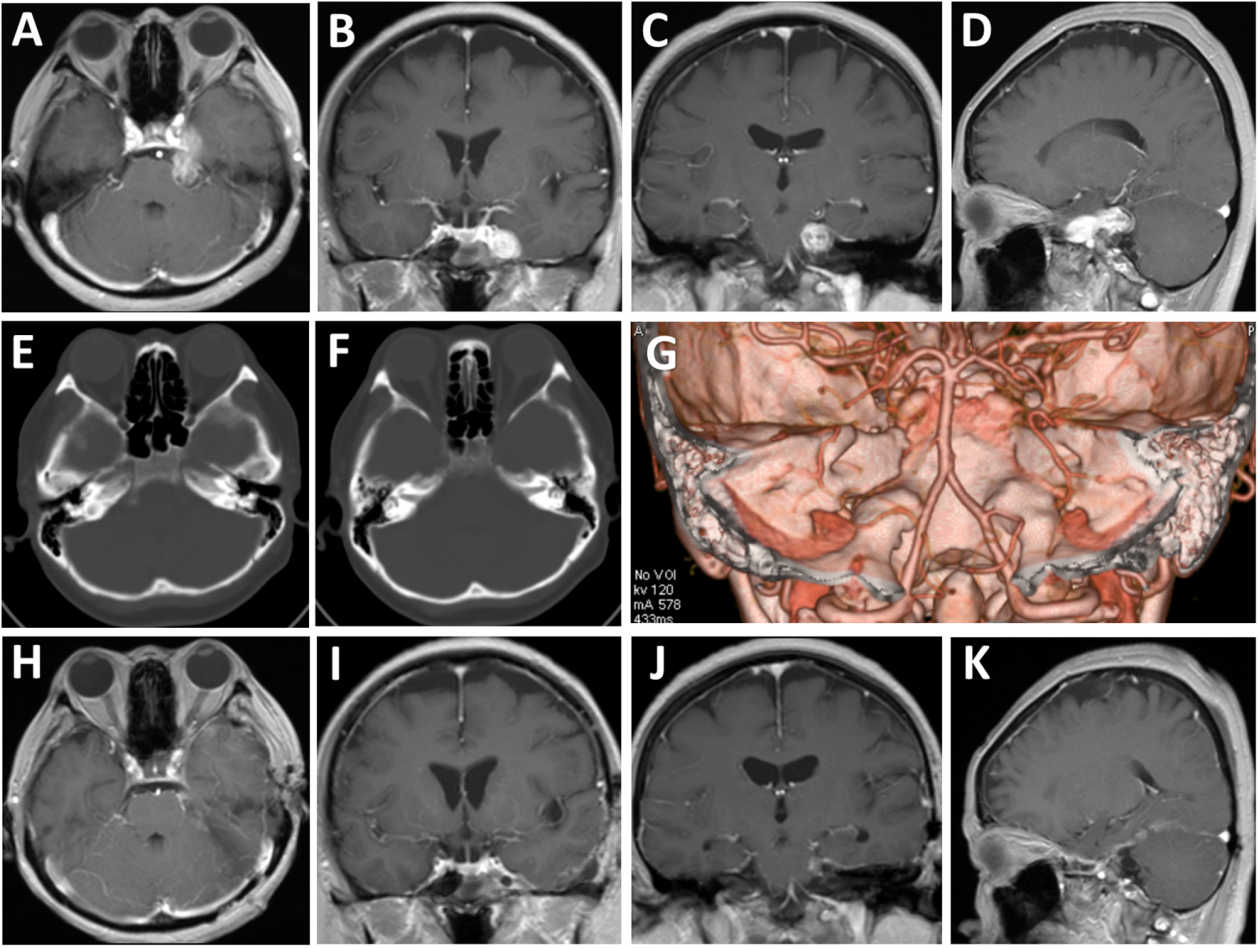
Radiological evaluation of the multi-compartmental lesion preoperatively and postoperatively. (A–D) Preoperative axial (A), coronal (B, C), and sagittal (D) T1-weighted magnetic resonance images with gadolinium enhancement. (E–G) Preoperative tomography images of bone windows (E, F) and three-dimensional reconstruction (G) revealed no erosion of the middle cranial fossa base and truncation of the petrous apex on the left. (H–K) Postoperative axial (E), coronal (F, G), and sagittal (H) T1-weighted magnetic resonance images with gadolinium enhancement.
